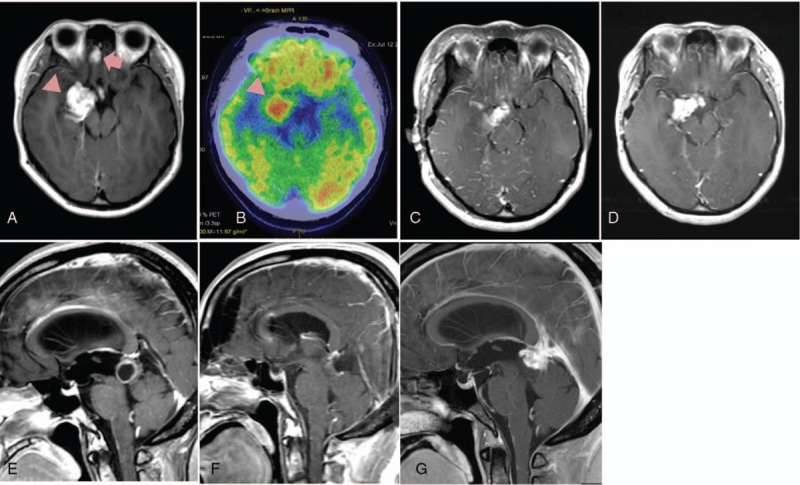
PET and gadolinium-enhanced MR imaging in case 1 (A–D) and case 2 (E–G). Case 1: Preoperative MRI shows two enhancing lesions in the frontal (A, arrow) and temporal lobes (A, arrow head), and PET imaging demonstrates high fluorodeoxyglucose (^18^F) uptake in the temporal lesion (B, arrow head). Partial removal of the tumors was confirmed by postoperative MRI (C), and MRI after adjuvant therapy revealed regrowth of the residual tumor (D). Case 2: Preoperative MRI shows a cystic tumor in the tectal plate (E). Complete resection of the tumor was confirmed by postoperative MRI (F). MRI after adjuvant therapy indicated local recurrence of the tumor (G).
